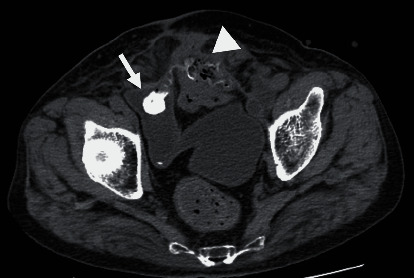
Abdominal computed tomography (CT) scan on arrival: the mesh migrated into the neobladder (arrow) and the small intestine (arrowhead).
